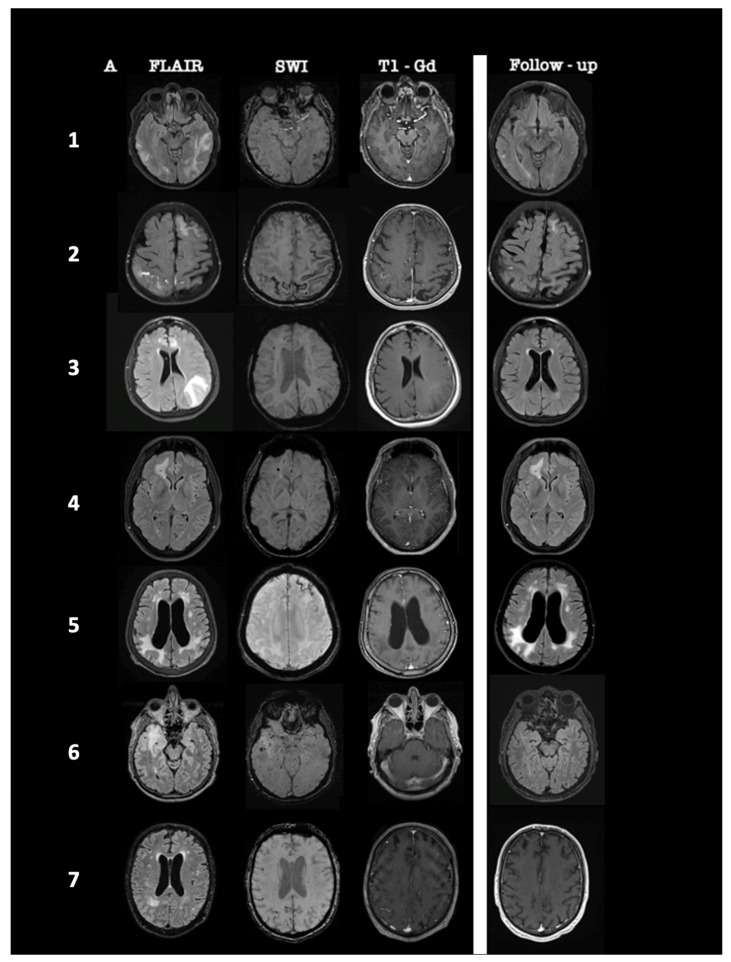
Summary of brain MRI characteristics of 7 patients at diagnosis and one month after treatment initiation. MRI findings of patients #1 to #7 are ordered from the top to the bottom. At diagnosis, axial fluid-attenuated inversion recovery (FLAIR) sequences showed supratentorial white matter lesions, multifocal in patients #1, #2, #3, and #5 and unifocal in patients #4, #6, and #7. In patients #1, #3, #4, #6, and #7, these lesions were associated with multiple cortical and subcortical cerebral microbleeds on Susceptibility Weighted Images, whereas patient #2 demonstrated left frontal cortical superficial siderosis (CSS) and subacute cortical subarachnoid hemorrhage (CSAH) on the right parietal lobe, and disseminated CSS was depicted on patient #5. White matter lesions on patients #2, #3, #4, #6, and #7 were associated with parenchymal or leptomeningeal contrast enhancement. In patients #1, #2, #3, #4, #5, and #6, follow-up axial FLAIR sequence one month after corticosteroid initiation showed a marked regression of hyperintense lesions on patients #1, #2, #3, and #6 and a stable size of hyperintense lesions on patients #4 and #5. In patient #7, the follow-up axial post-gadolinium T1 sequence revealed a complete resolution of gadolinium enhancement.
